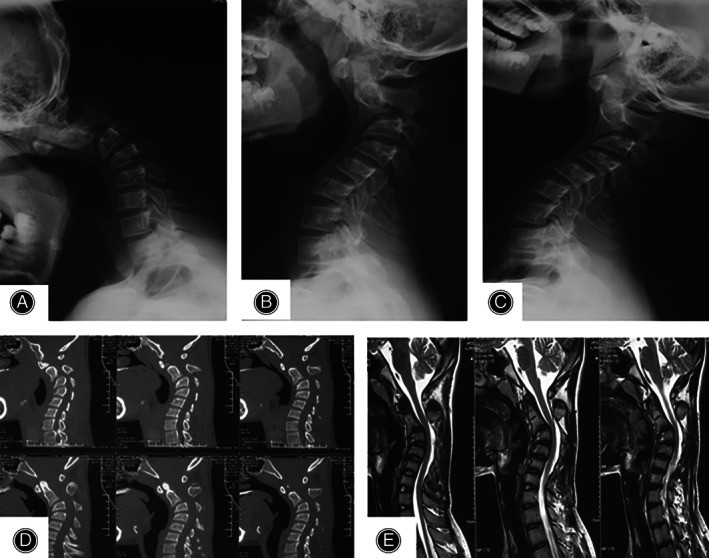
Preoperative radiology: lateral flexion(A), neutral(B), and extension(C) showing a cervical kyphotic deformity. Sagittal CT scan(D) showing the kyphotic bony anatomy with no sign of osteophyte formation. Sagittal MRI(F) showing no obvious cord compressing.
